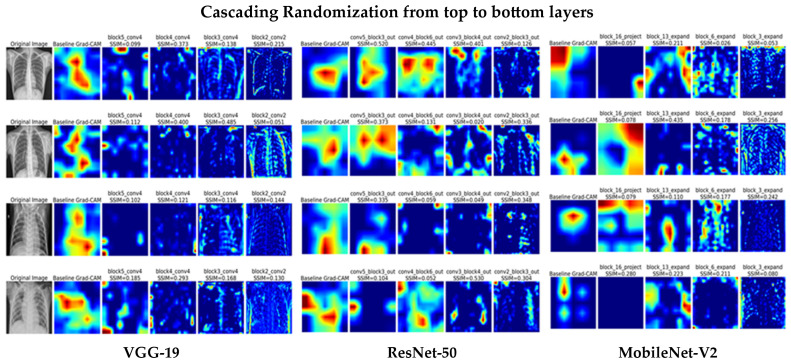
Grad-CAM sanity check: attention maps from VGG-19, ResNet-50, and MobileNet-V2 with cascading model randomization on normal and pneumonia chest X-rays.
